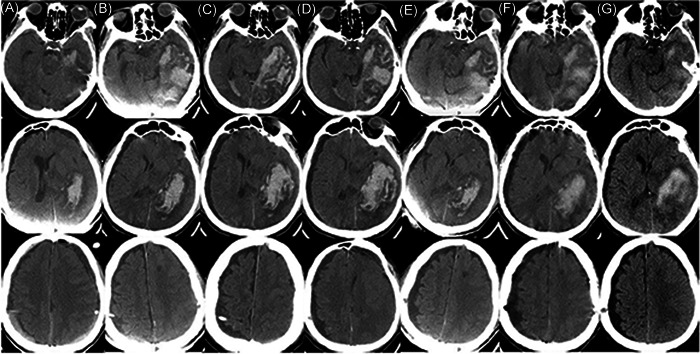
Postoperative brain computed tomography (CT) showed hemorrhage in the temporal lobe, occipital lobe, and the occipital angle of the right lateral ventricle. Columns (A–G) represent cranial CT immediately after the operation and on days 2, 3, 4, 5, 11, and 19 after the operation, respectively
